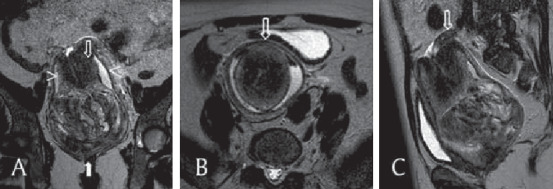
T2-weighted MRI of complete nonpuerperal uterine inversion due to fibroid. (a) This coronal image shows a vaginal heterogeneous mass (filled arrow), with the uterine corpus in a U-shape above the mass (empty arrow). The cervix surrounds the corpus, and the vaginal fornix surrounds both the corpus and the cervix (arrowheads). (b) This axial image shows, from the center outwards, the uterine corpus, the cervix, and the fornix and the invaginated round ligaments in a bullseye appearance (arrow). (c) This sagittal image shows one ovary above the cervix (arrow) [74].
